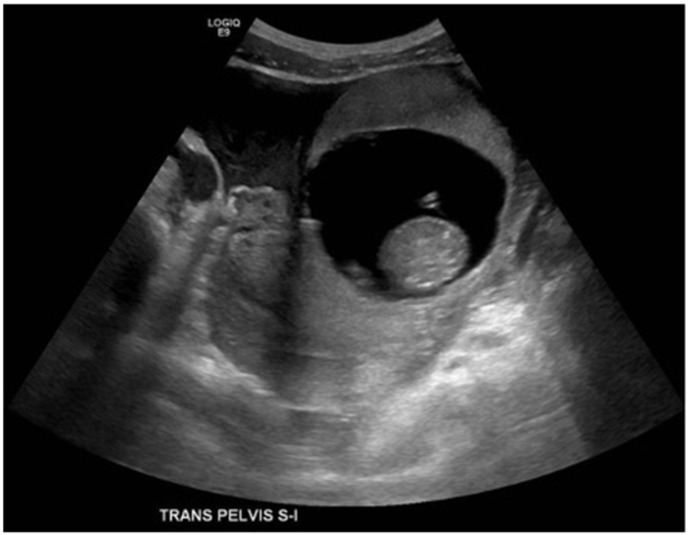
A 33-year-old female presented with diffuse abdominal pain. Transverse ultrasound of the pelvis through the level of the uterus demonstrating gestational sac outside of the uterus and free pelvic fluid. Estimated live extrauterine gestational age of 13 weeks 6 days +/− 1 week 2 days. It is unclear whether the ectopic pregnancy is located within the left fallopian tube or involves the left ovary.
